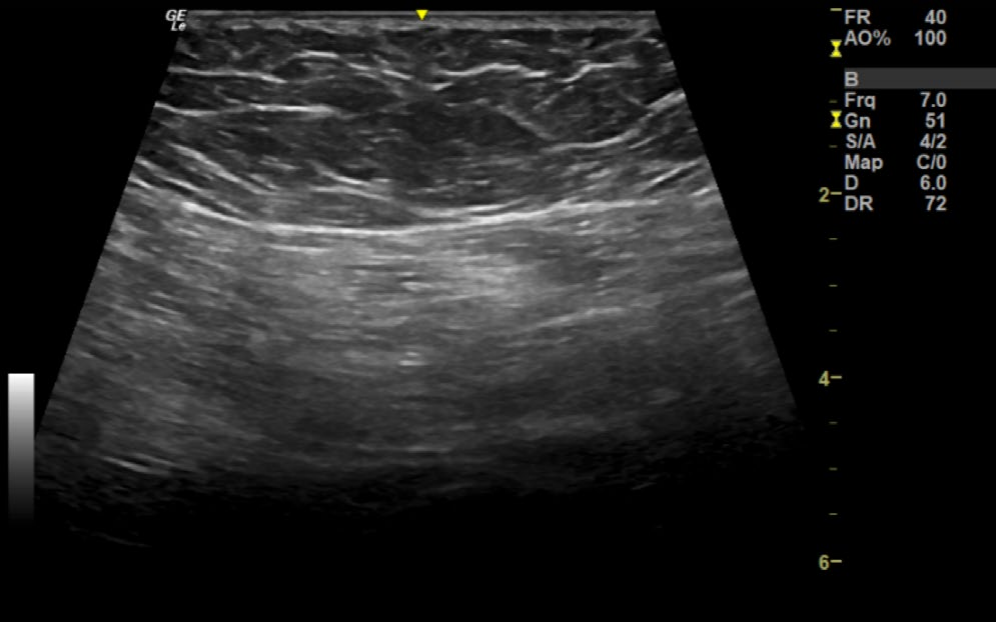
Intramuscular PMMA in the gluteus maximus observed in this image in the longitudinal axis with a linear transducer (6–15 mHz). Preserved subcutaneous tissue with a hypoechoic ultrasound pattern with hyperechoic lines (usual pattern of subcutaneous tissue without fillers). The echogenicity of the musculature (below the muscle fascia) is increased and there is a loss of the usual fibrillar pattern.
